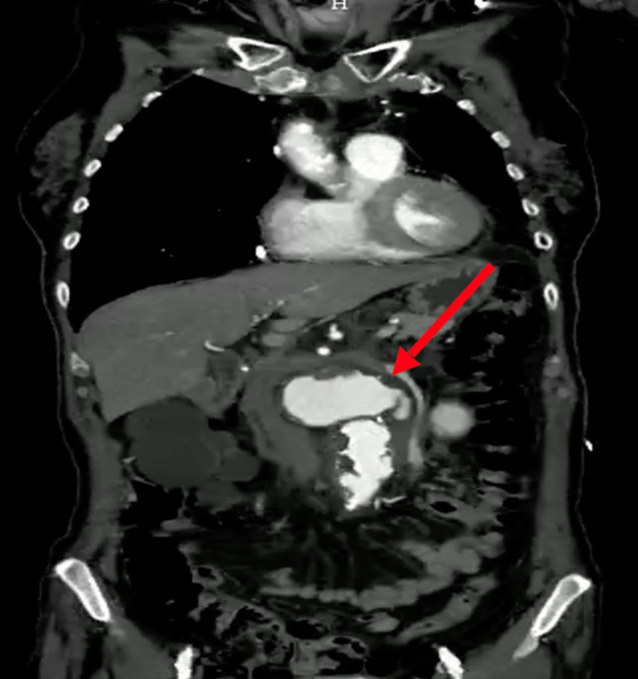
Ausgangsbefund des infrarenalen BAA mit retroperitonealer Perforation – CT-Aufnahme des Abdomens (koronare Rekonstruktion; Fall 1). Der *rote Pfeil* markiert das BAA (Quelle: Klinik für Radiologie und Nuklearmedizin, Universitätsklinikum Magdeburg A. ö. R.)
